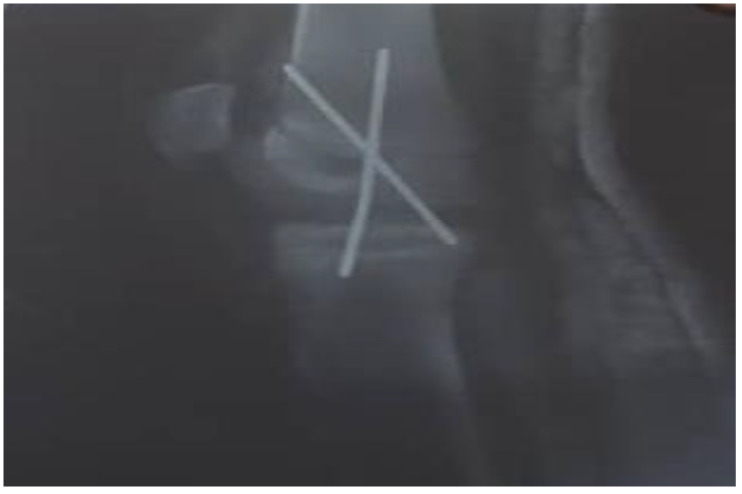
Plain X-ray of the left knee with percutaneous cross-pins for bone stabilization.
